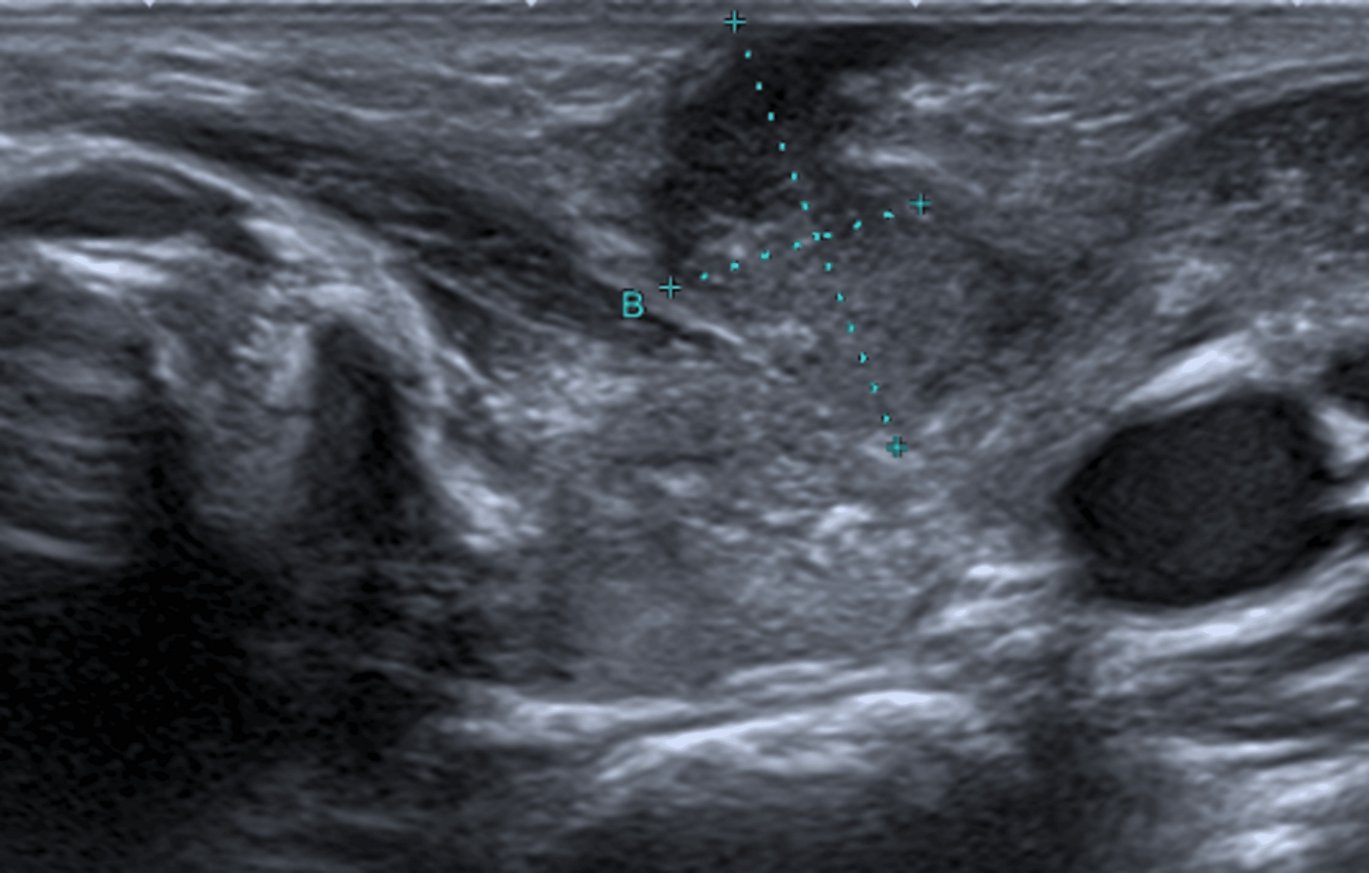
Thyroid ultrasound revealing a tortuous fistulous tract (12×7 mm) with a sinuous course extending toward the skin surface, indicative of external fistulization.
